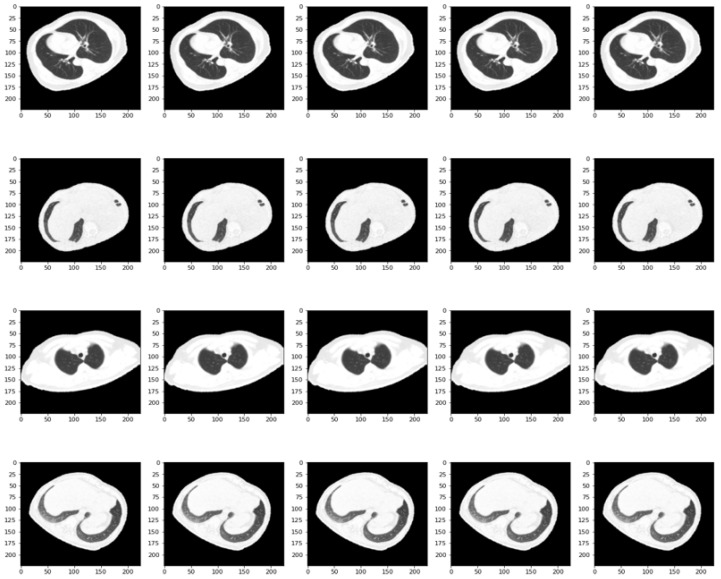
A batch of 20 samples from the resized and augmented COVID-19-CT dataset.
